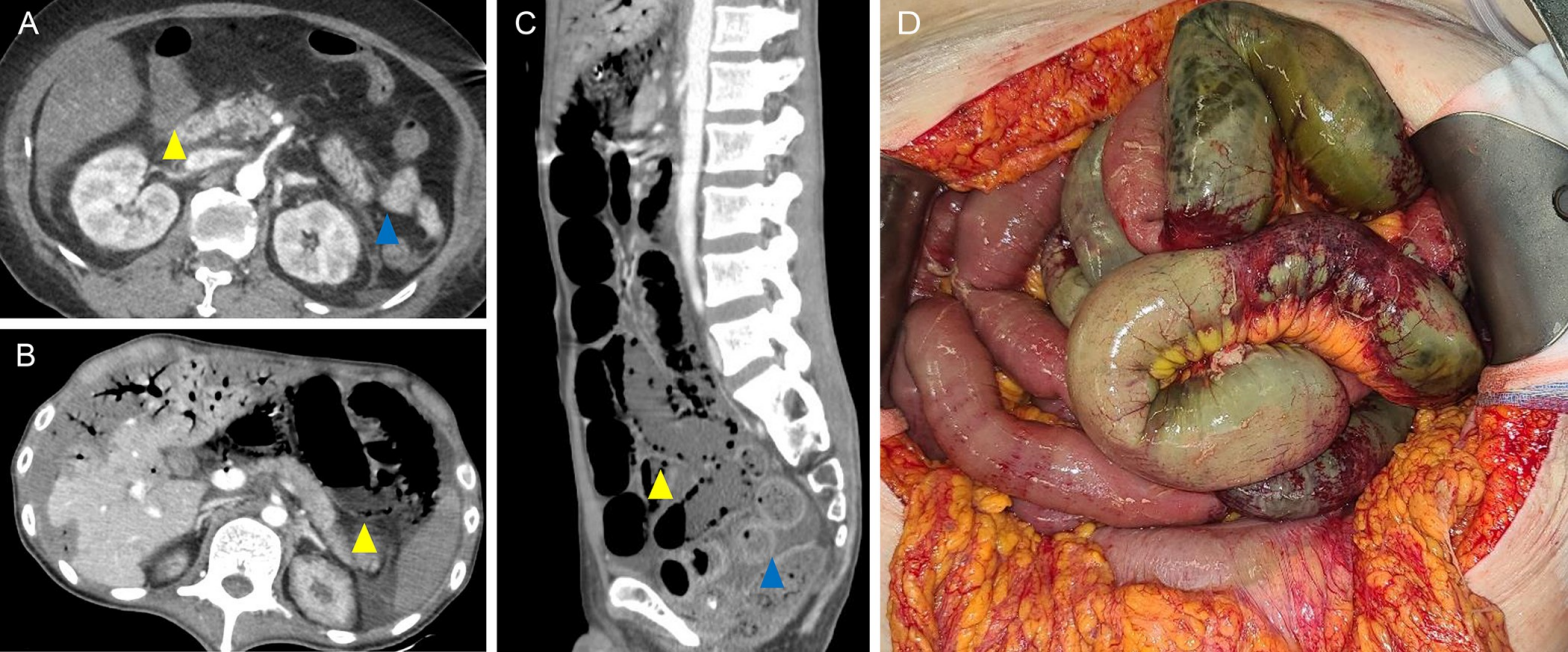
Contrast-enhanced CT images and intraoperative bowel images of non-occlusive mesenteric ischemia. (A) Contract-enhanced abdomen CT images of survivor, transverse image (B) Contract-enhanced abdomen CT images of non-survivor, transverse image (C) Contract-enhanced abdomen CT images of non-survivor, sagittal image (D) intraoperative bowel images; multiple segmental bowel necrosis was observed, but also normal bowel and mesentery found between necrotic bowel segments. Yellow arrows indicate the dilated and thinned bowel and blue arrows indicate normal bowel.
